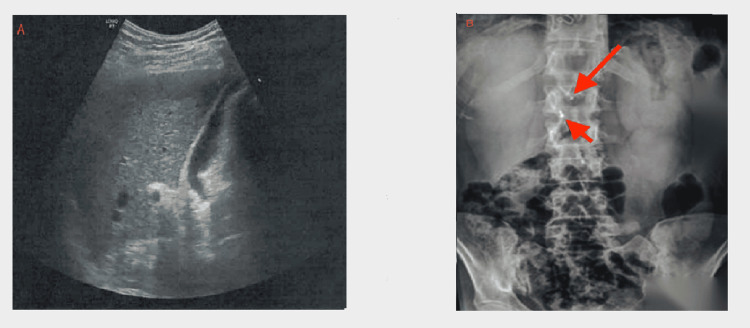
(A) Ultrasound showing normal liver parenchyma without biliary dilatation. (B) Plain radiography showing post-cholecystectomy clips (small arrow) and a linear radiopaque tubular structure (large arrow) in the right upper quadrant
